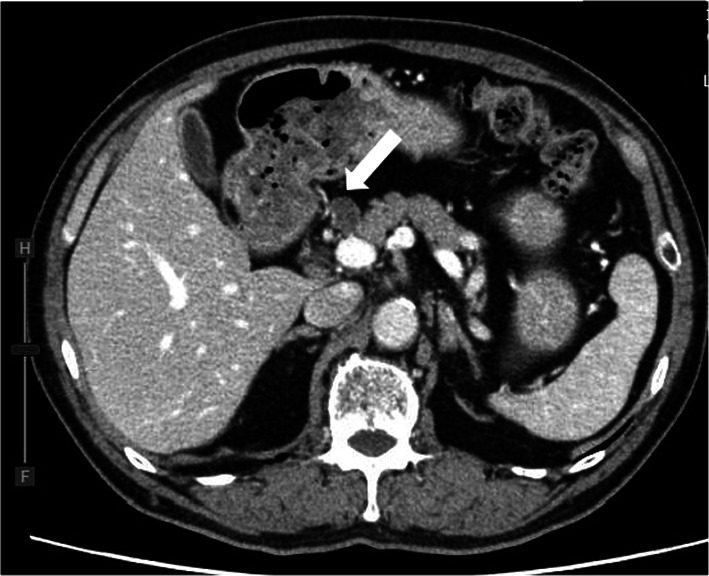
Radioiodine whole-body scintigraphy demonstrated uptake of I-131 in the thyroid bed and the pancreas head (white arrow)
